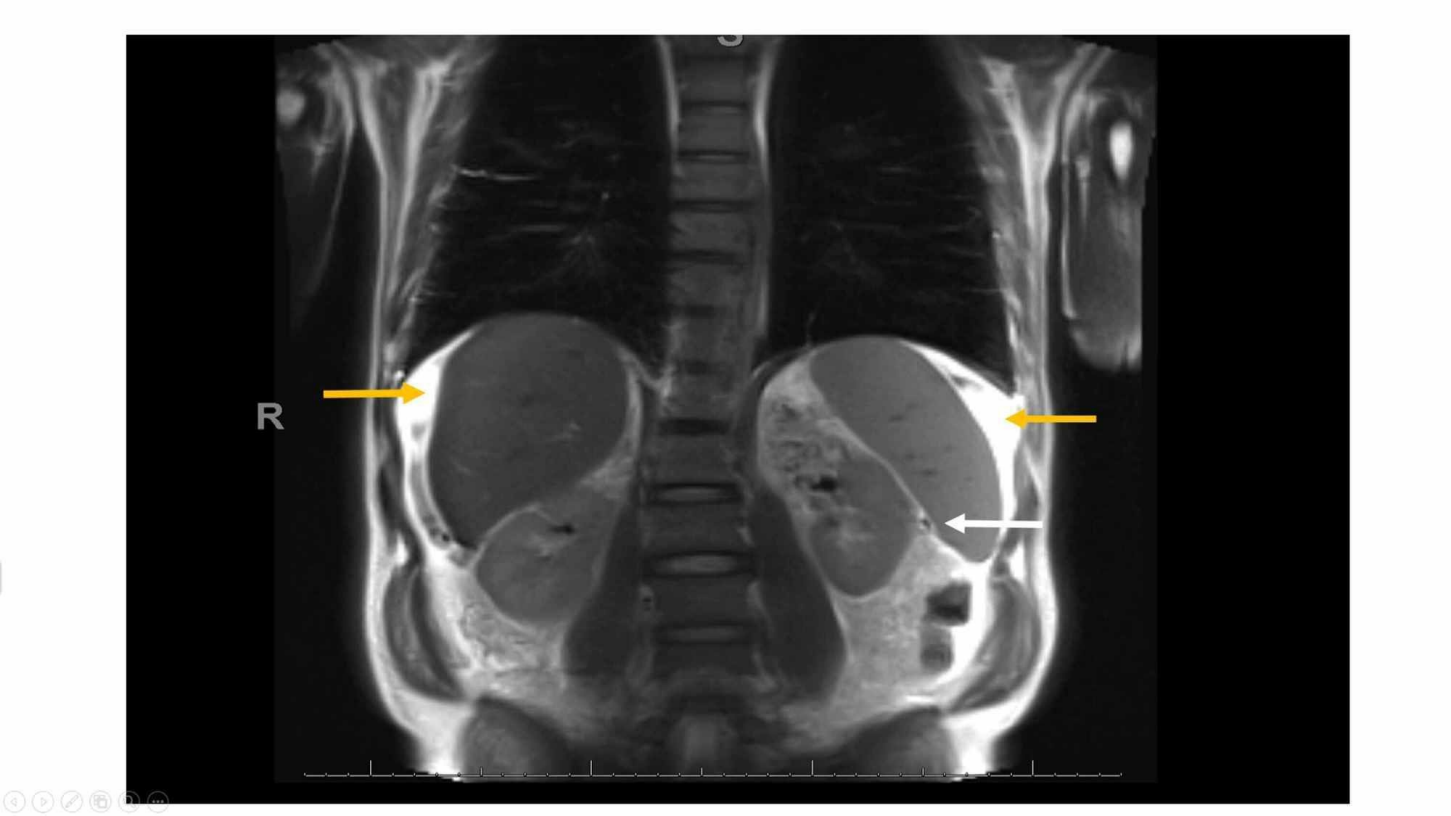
MRI and magnetic resonance cholangiopancreatography of the abdomen MRI showing ascites around liver and spleen (yellow arrows), and splenomegaly (white arrow).
